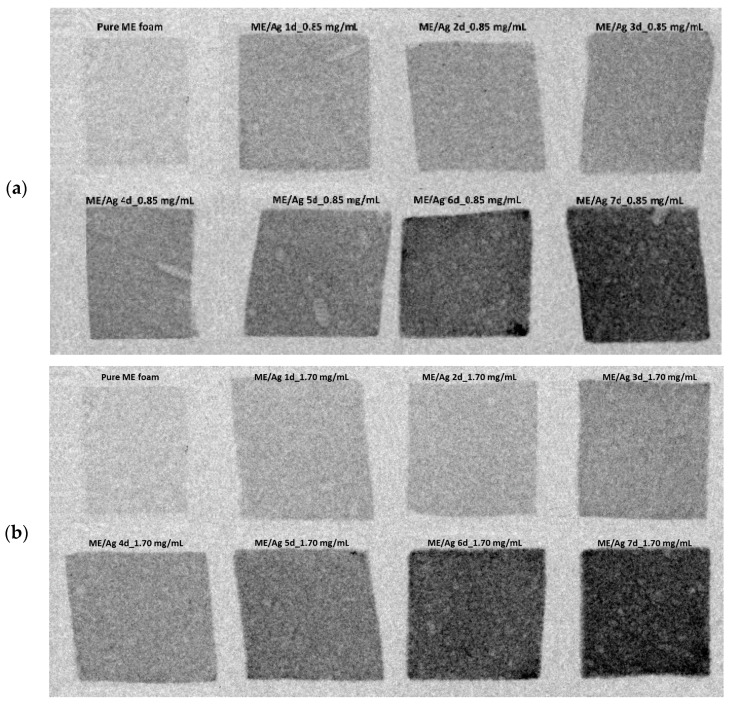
X-ray radioscopy of the untreated ME (pure) and functionalized ME/Ag using 0.85 mg/mL (a) and 1.70 mg/mL (b) as solution precursor for different reaction times from 1 to 7 days.
